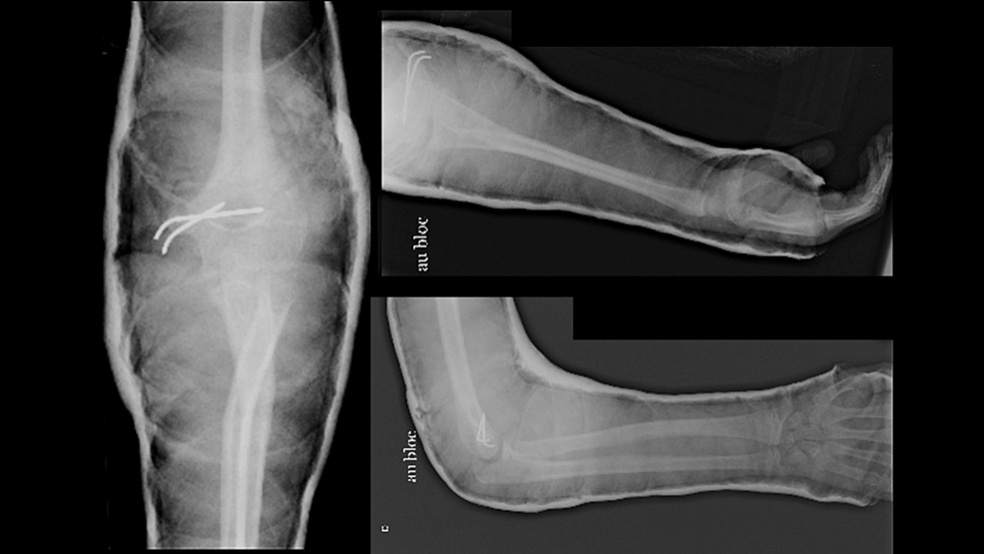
Radiographs of the elbow after open reduction internal fixation.
